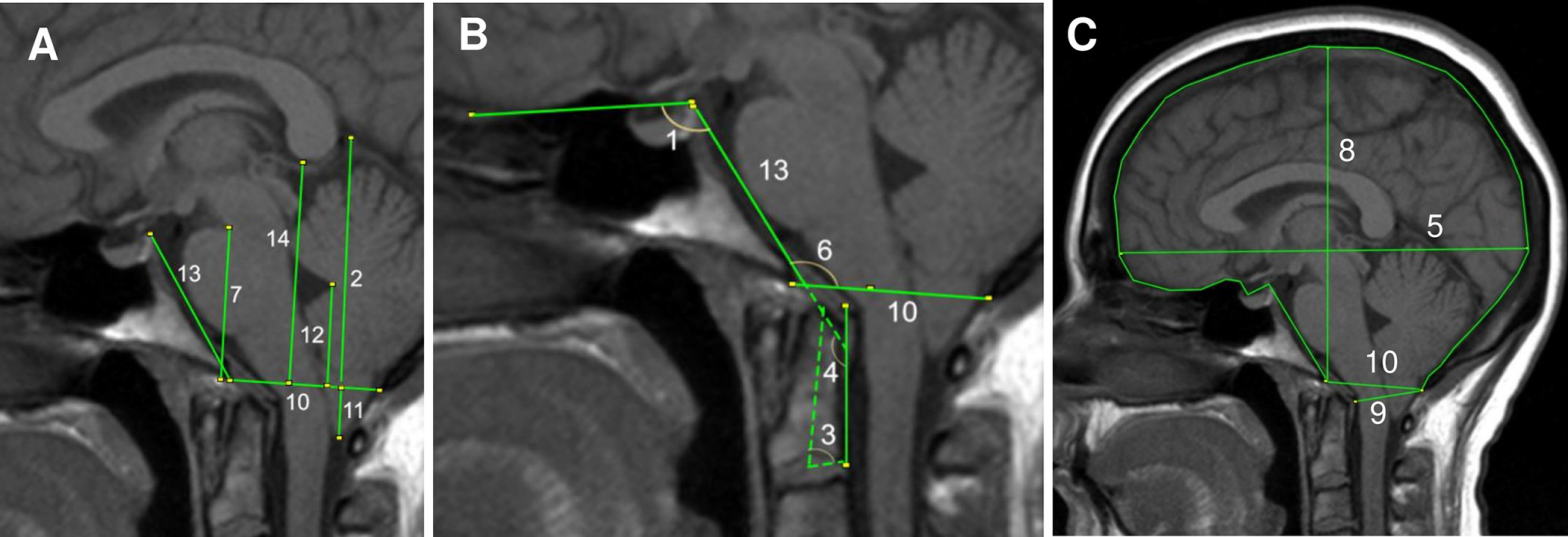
Fourteen morphometric measurements taken on a midsagittal T1-weighted MRI. A: 2-Posterior cranial fossa height, 7-pons height, 10- McRae line length, 11-tonsillar position, 12- fastigium height, 13- clivus length, 14-corpus callosum height. B: 1-Basal angle, 3-odontoid angle, 4-Wackenheim angle, 6- Boogard angle. C: 5-intracranial diameter, 8- intracranial height, 9-anteroposterior diameter dura-opisthion.
